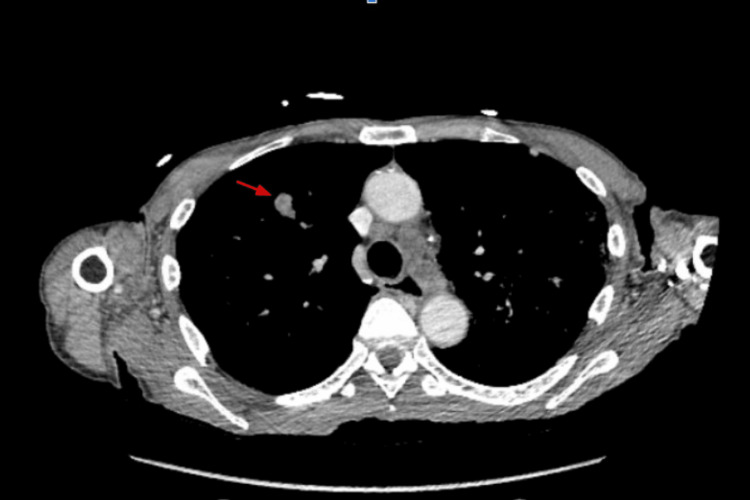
CT of the chest with contrast (axial view): pulmonary nodule likely representing metastatic disease (red arrow)
